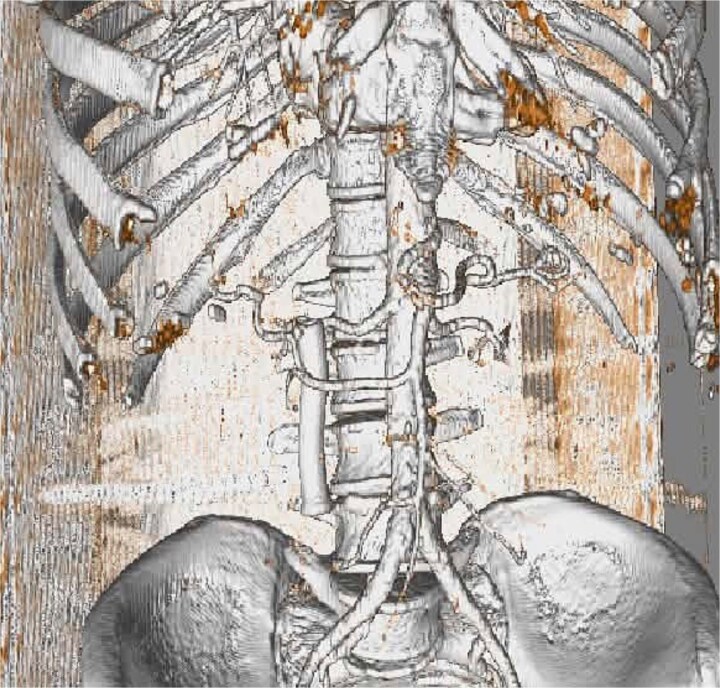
3D CT angiography reconstruction showing the solitary RHA arising from the SMA and coursing through the pancreatic parenchyma. This reconstruction confirms that the RHA is the sole arterial supply to the liver.
